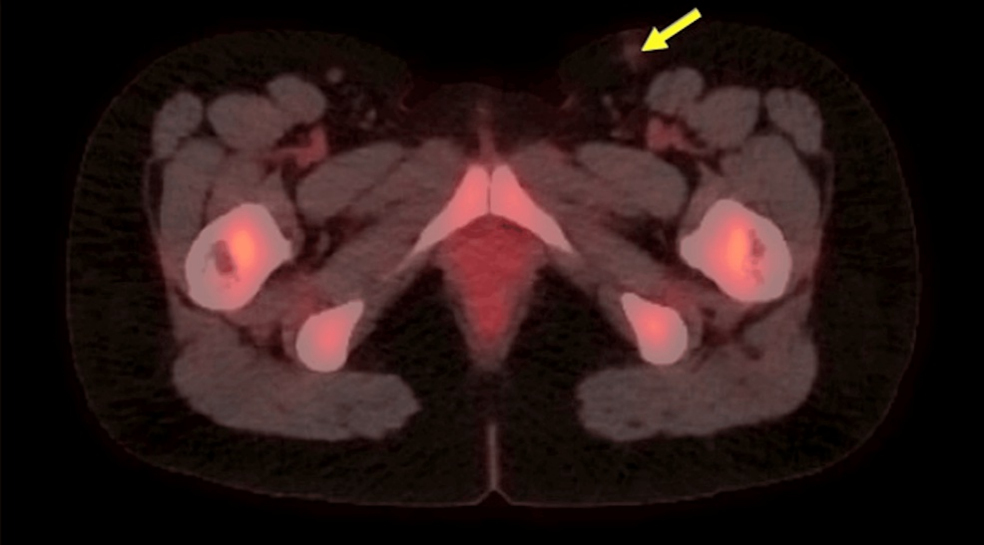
Axial PET/CT imaging demonstrated no evidence of new, persistent, or recurrent malignant disease. A previously FDG-avid lymph node in the left inguinal region has resolved nearly to the background (blue arrow) PET/CT: positron emission tomography/computed tomography; FDG: fluorodeoxyglucose
